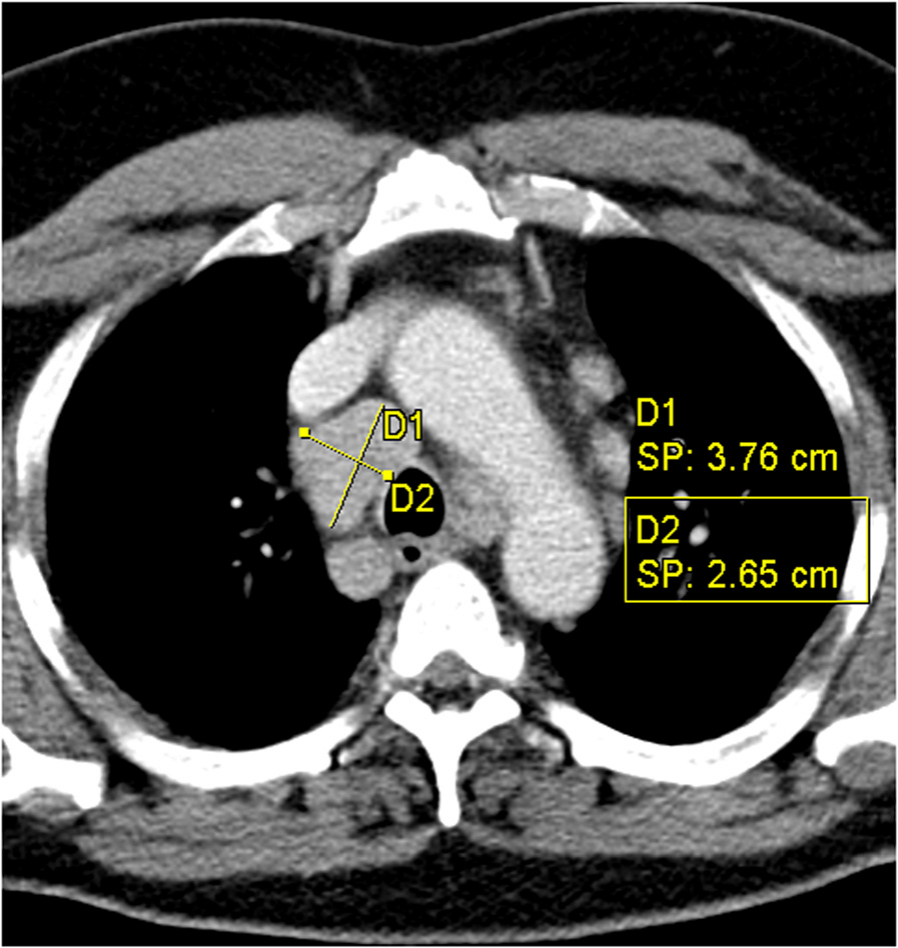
Example of an abnormal mediastinal lymph node. An axial image of a contrast-enhanced computed tomography examination of the thorax. The size of a lymph node is specified by measuring the longest in-plane diameter and the corresponding short axis in the orthogonal direction
